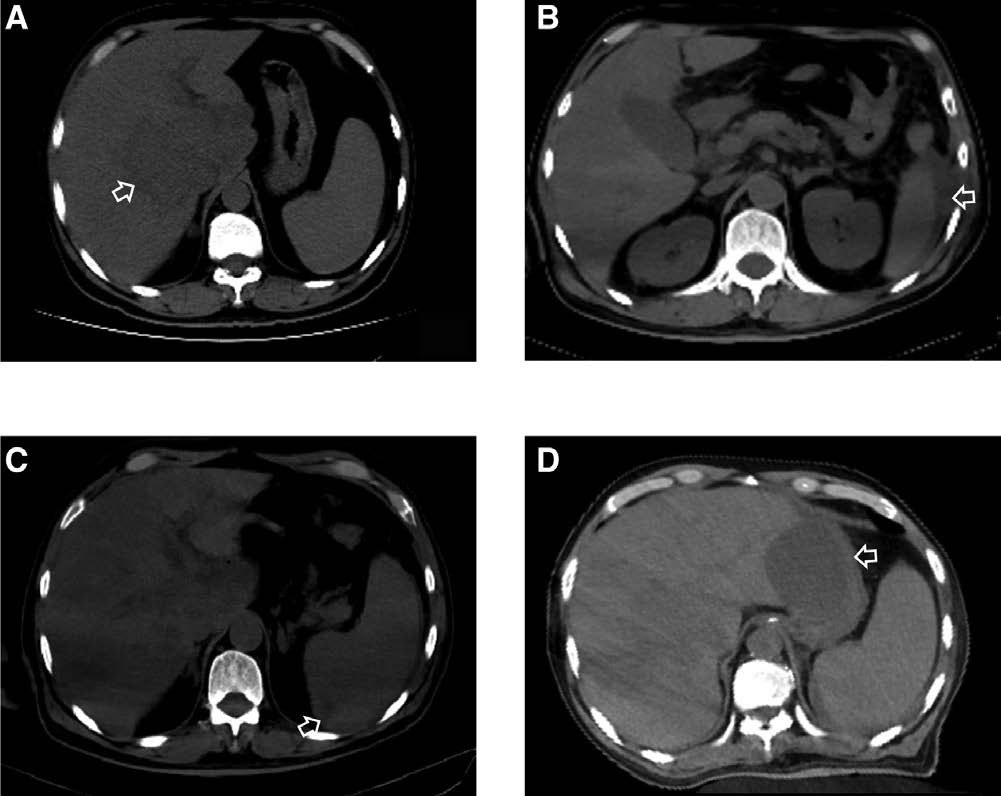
(A) Chest CT showed liver abscess before admission. (B and C) After 4 days in ICU, perisplenic and splenic abscess occurred. (D) After 8 days in ICU, abdomen CT showed cystic low-density shadow around the gastric wall, considering abscess liquefaction. CT = computed tomography, ICU = intensive care unit.
